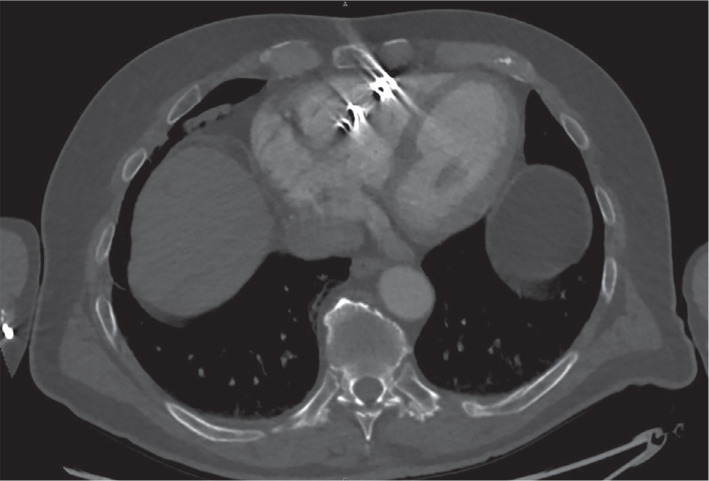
Visualization of the Nanostim leadless pacemaker on a computed tomography scan of the chest. The patient’s preoperative chest computed tomography scan showed that the previously implanted Nanostim leadless pacemaker was positioned in the lateral free wall of the right ventricle, adjacent to the tricuspid annulus.
